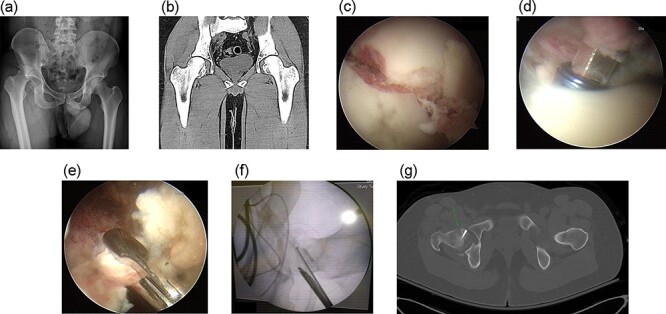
A 28-year-old man (Case 4) with a Pipkin Type I fracture of the right hip with posterior dislocation of the femoral head. (a) The anteroposterior X-ray image of the injury in the right hip. (b) The pre-operative anteroposterior CT scan of the right hip after closed reduction showing inferior mini displacement of the femoral head fragment. (c) Fluoroscopy showing fracture reduction of the femoral head after guidewires insertion and (d) after fixation of screws during the surgery. (e) Concomitant intra-articular hip lesions secondary to traumatic hip dislocation can also be treated. (f) Fluoroscopic examinations confirmed the successful and secure fixation of the fracture fragments without protrusion. (g) The post-operative CT scan showing acceptable internal fixation and congruity of the femoral head ).
